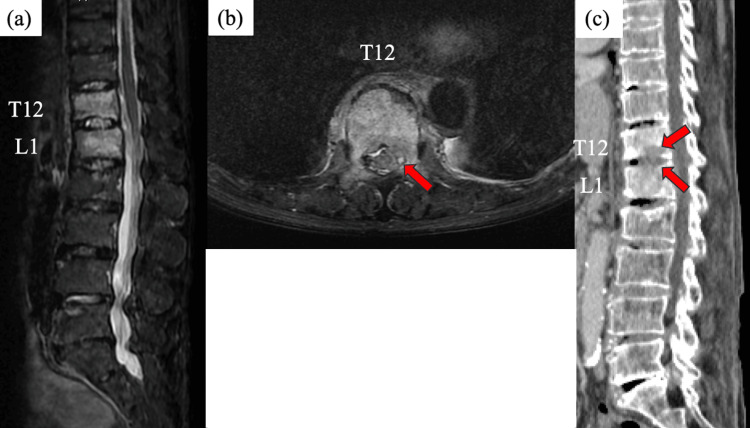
Image examination (a) The sagittal section of the spinal MRI on day one revealed high-signal alterations consistent with pyogenic spondylitis in the T12 and L1 vertebrae. (b) The axial section of the spinal MRI at the T12 level on day X+1 revealed a high-signal region within the spinal canal, suggestive of an epidural abscess. (c) The sagittal section of the CT scan on day X-5 revealed osteolytic alterations in the T12 and L1 vertebral bodies CT: computed tomography; MRI: magnetic resonance imaging
